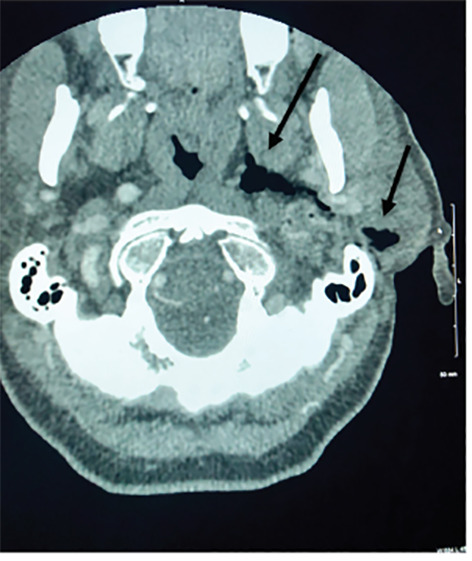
Computed tomography demonstrating soft tissue injury with subcutaneous air extending from the external acoustic meatus to the carotid space (arrows).
